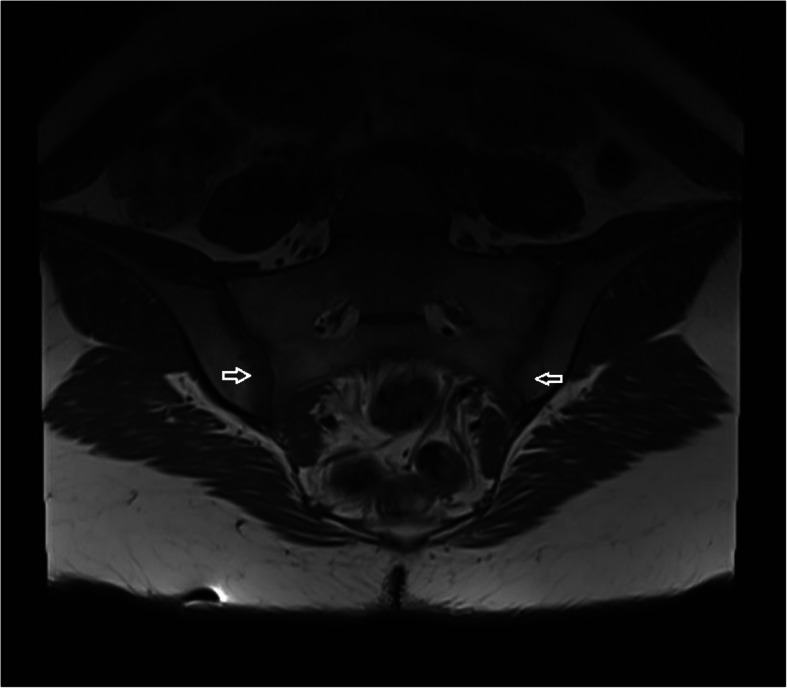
Semicoronal T1-weighted spin-echo image shows signal loss (arrows) in sacroiliac joints consistent with sacroiliitis
